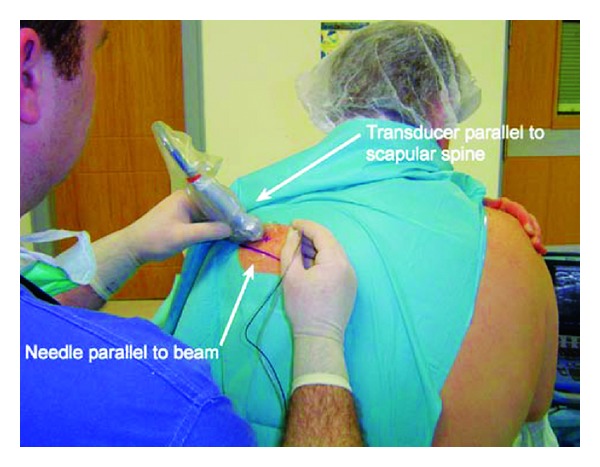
Ultrasound probe and needle position for suprascapular nerve block (Harmon and Hearty [6]). (Reprinted with permission.)
